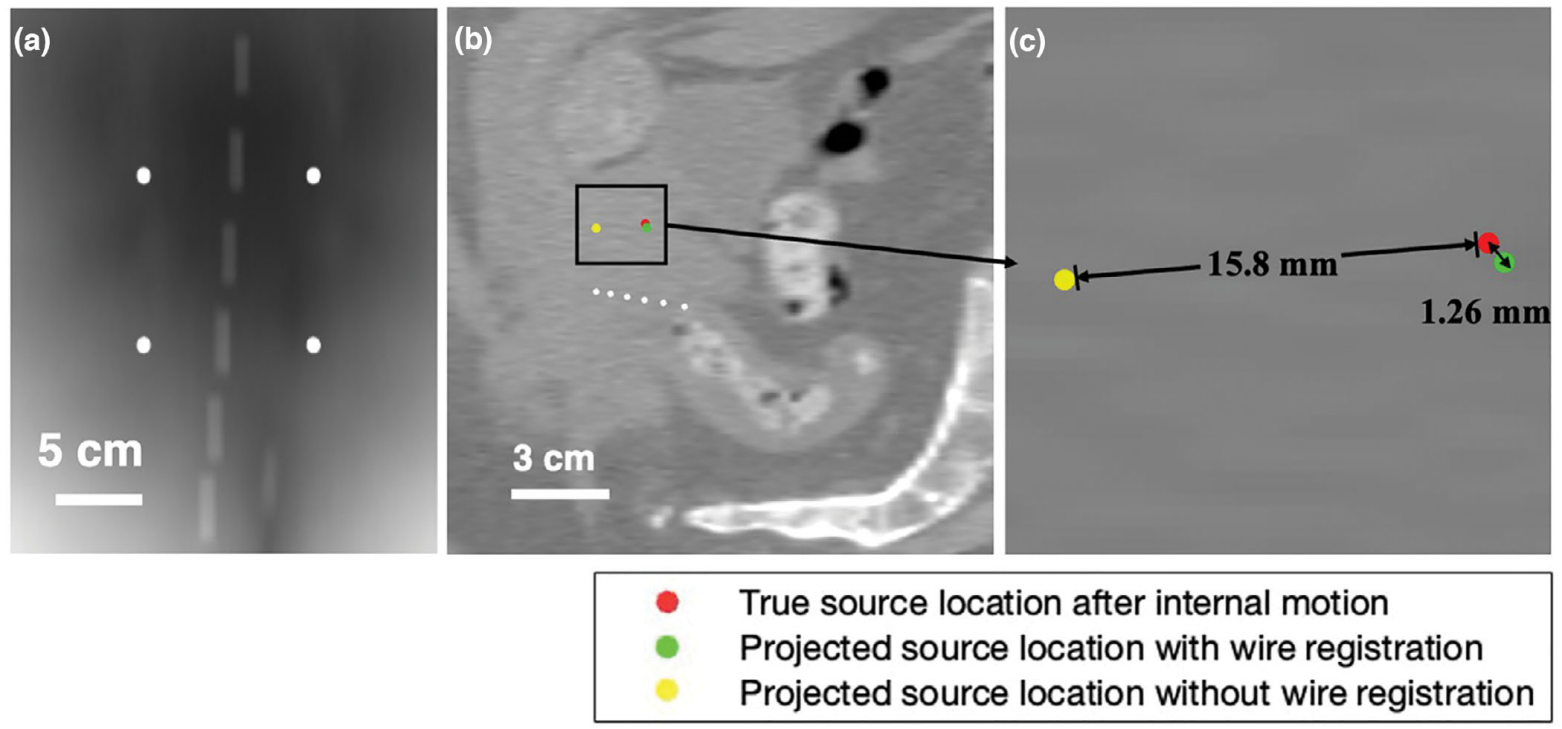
(a) Projection image of tilted wire from ray tracing. (b) Contrast‐adjusted CT slice sagittal image showing wire‐BBs, source location, and projected source locations. (c) Zoomed in view highlighting position deviations.
